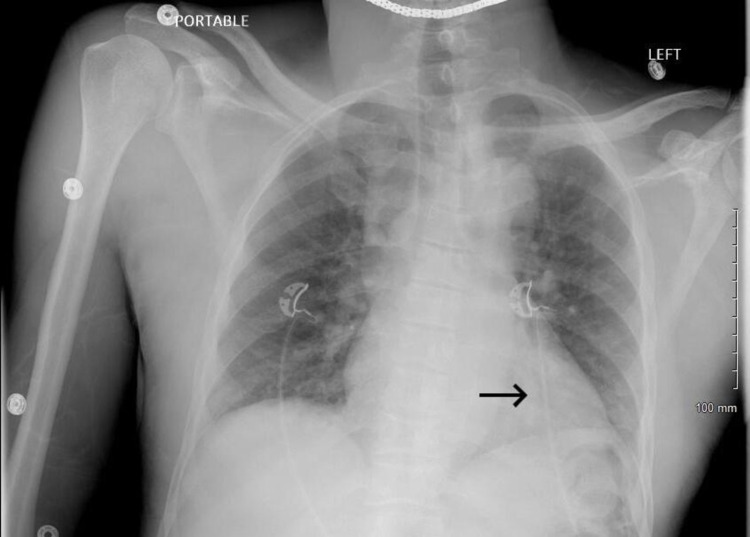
Chest X-ray showing reticular densities with patchy alveolar opacities in the left lung base/retro cardiac region (suggesting developing pneumonia)
